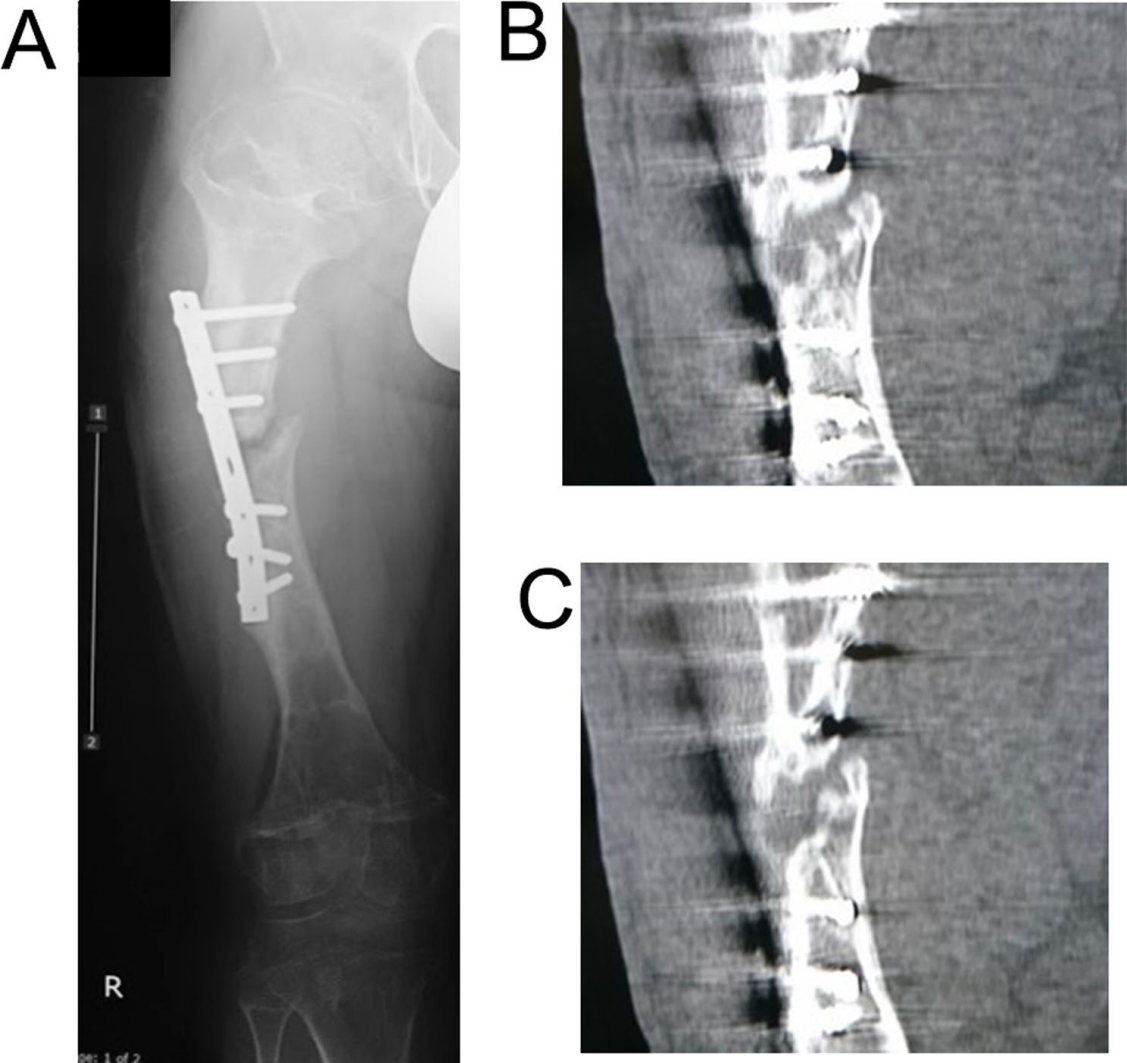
X-ray (A) and CT (B, C) show osteomyelitis and pathological fracture by deep pin-tract infection at the femur
